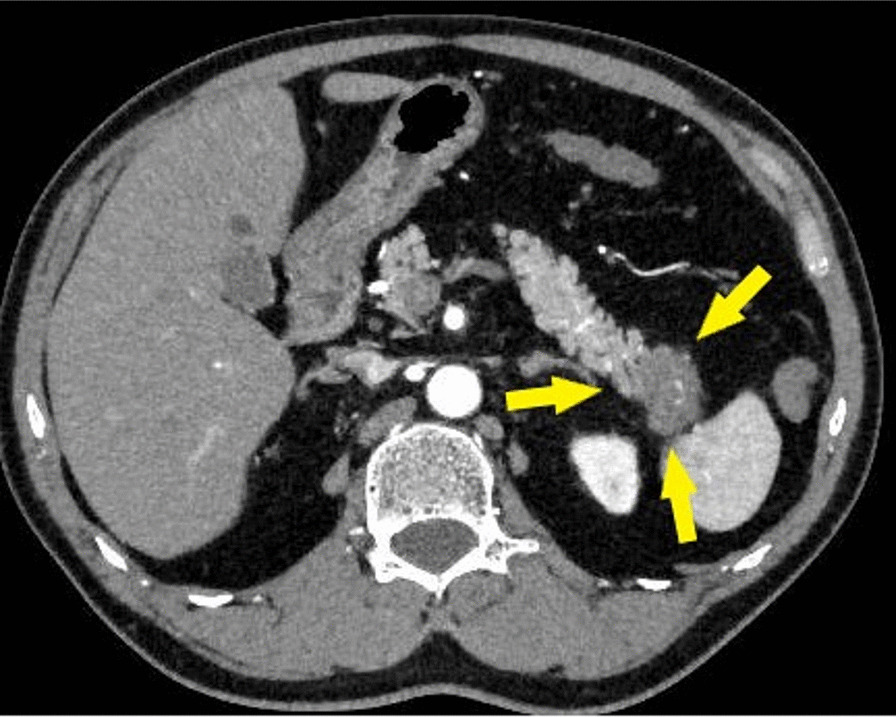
Preoperative contrast-enhanced computed tomography scan. The yellow arrow indicates a pancreatic cancer site
